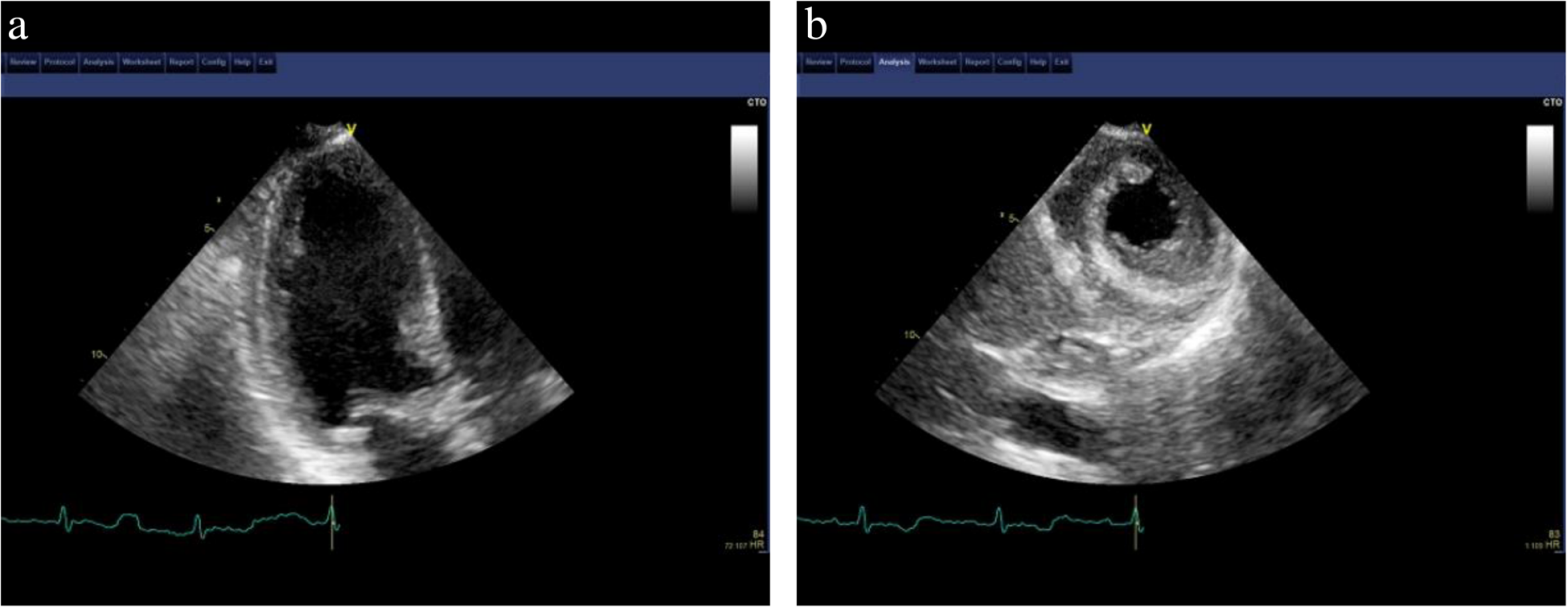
Transthoracic echocardiographic images. Long-axis (a) and short-axis (b) views obtained after 1 week of anticoagulant treatment showing complete resolution of the apical thrombus
